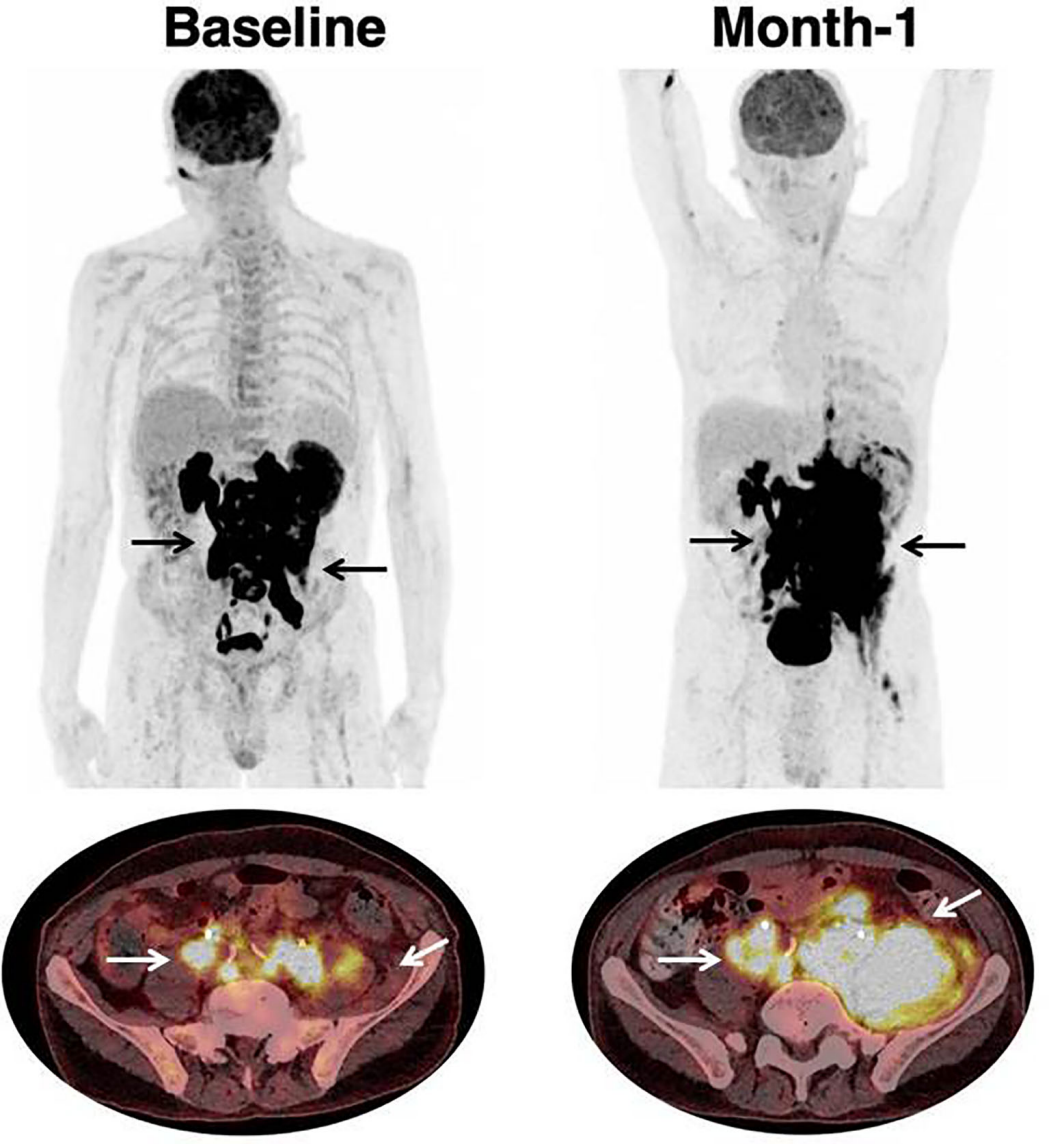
Progression in a patient treated with CAR T-cell therapy. 68 year-old patient with past medical history of DLBCL diagnosed one year prior to treatment initiation. Patient had Stage IV disease, with rearrangement of the MYC and BCL6 genes, and treated with two prior lines of chemotherapy. Black (on Maximum Intensity Projection) and white arrows (on axial fusion images) show infradiaphragmatic lymphadenopathies, with muscular infiltration. Baseline imaging showed high tumor volume which is typically associated with unfavorable outcome and lower response rate to CAR T-cell therapy. Follow-up imaging showed a progression on CT-scan as well as on [18F]-FDG PET. At month-1, there were new lesions as well as an increase in tumor volume. The prognosis was poor; hence salvage treatment and later best supportive care were initiated. Patient died at month-2.
